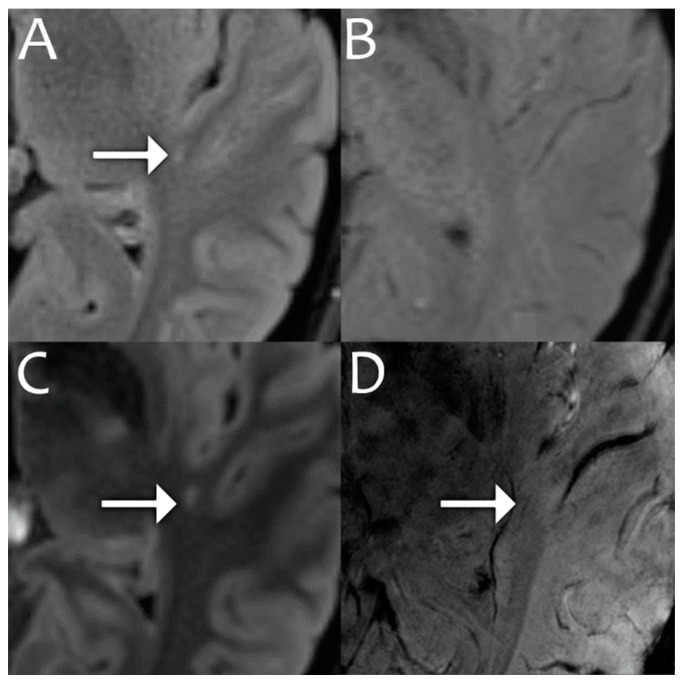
3T FLAIR (A) demonstrates a hyperintense lesion (white arrow) with no definite central vein on 3T SWI (B). 7T FLAIR (C) demonstrates a hyperintense lesion (white arrow) with a corresponding central vein on 7T SWI (D), which is more suggestive of an MS demyelinating lesion. Images acquired at the Mayo Clinic.
